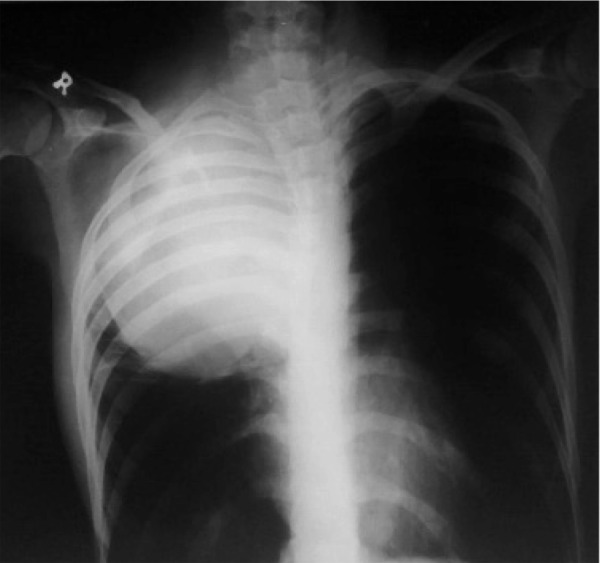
A 13-year-old boy with apparent deformity of the spinal column and recent dyspnea. PA chest X-ray.
